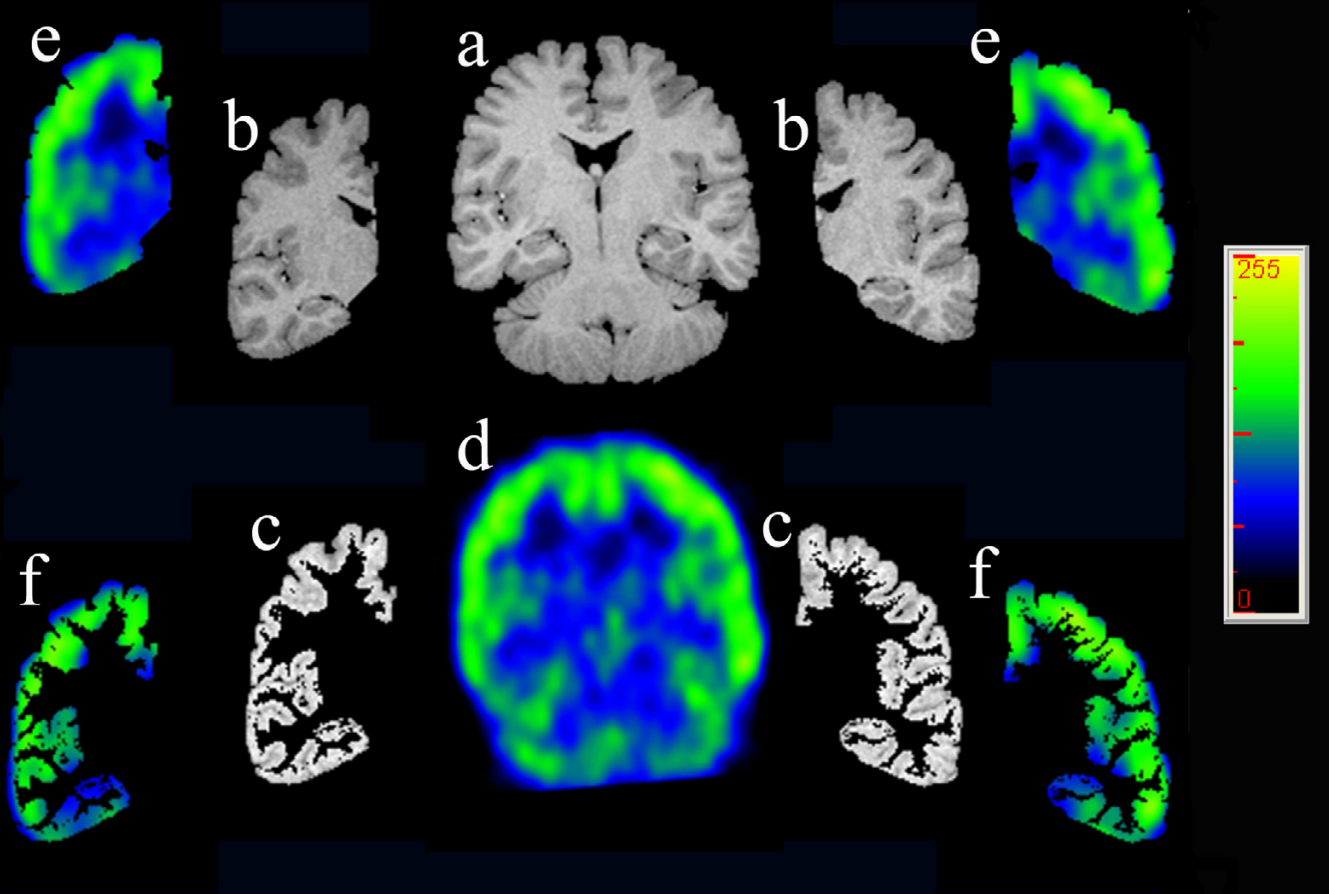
Volumetric MRI and coregistered FDG-PET: (a) MRI Extracted whole brain is segmented into (b) MRI right and left cerebral hemispheres, in turn thresholded into (c) MRI right and left cerebral cortices. Coregistration of the (d) FDG-PET whole brain allows accurate anatomical definition on the metabolic studies of, for example, (e) FDG-PET right and left cerebral hemispheres and (f) FDG-PET right and left cerebral cortices.
